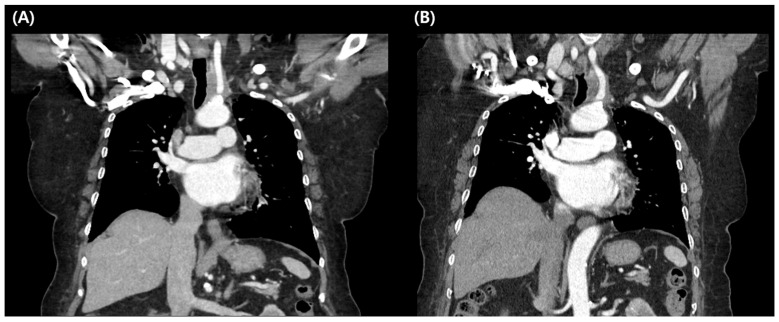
Comparison of serial chest computed tomography (CT) images before and after treatment. (A) Pretreatment chest CT; (B) chest CT obtained 14 months after atezolizumab treatment. The infiltrative enhancing mass in the left perithyroidal region shows stable disease.
